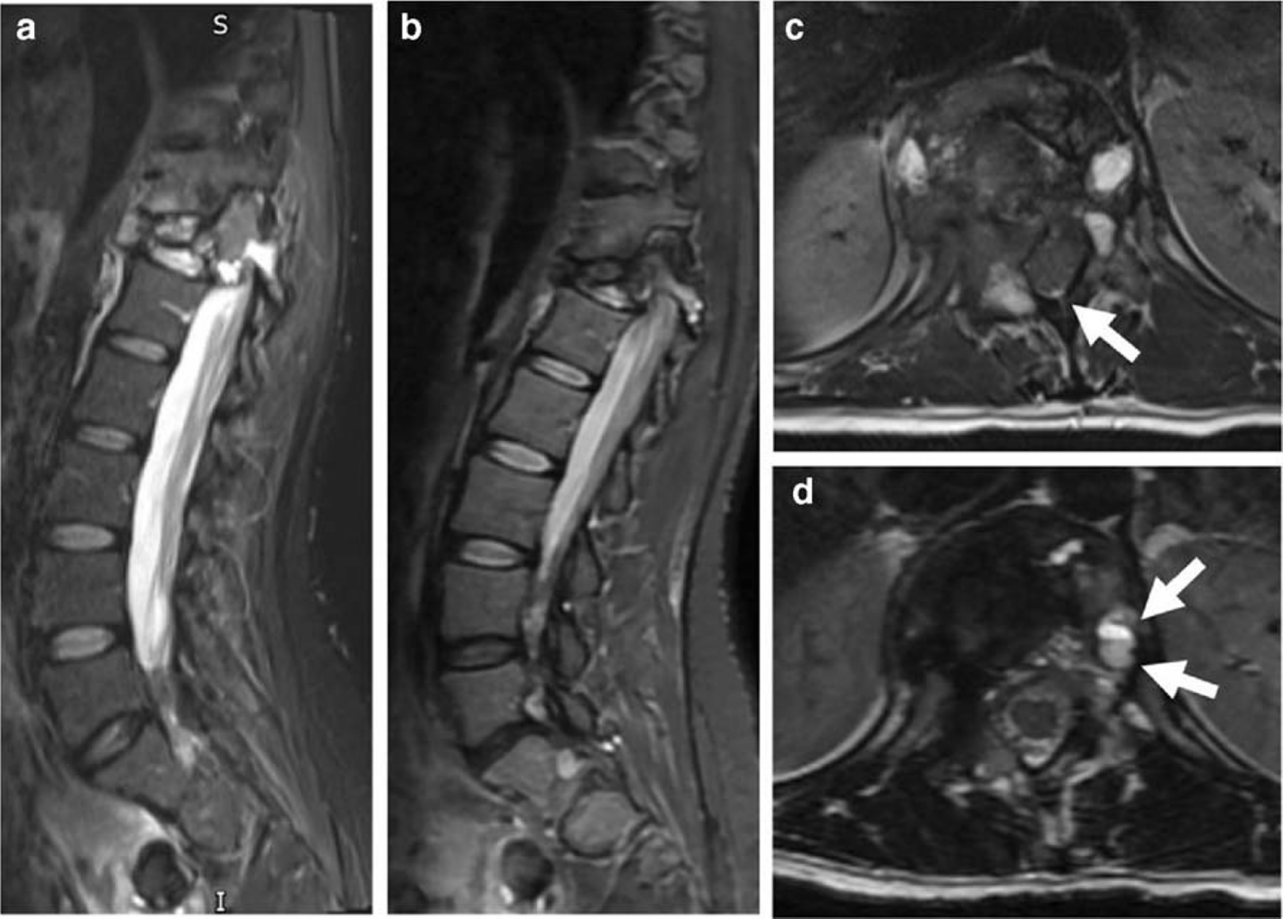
A 30-year-old female presented with back pain and a newly developed scoliosis. MRI at presentation (a, c) and after 8 weeks on denosumab (b, d). a Sagittal T2 TIRM MR image at baseline shows T12 vertebral collapse secondary to a GCTB with heterogeneous signal intensity and cystic components. b Sagittal T2 TIRM MR image after 8 weeks on denosumab demonstrates decreased size and signal intensity of the tumour, with further loss of height of the vertebral body. c Axial T2-weighted MR image at presentation shows tumour extension into the paravertebral soft tissues and epidural space. There is almost complete obliteration of the spinal canal with minimal remaining CSF posteriorly (white arrow). d Axial T2-weighted MR image after 8 weeks on denosumab shows decreased size and signal intensity of the tumour, with a more defined low signal margin and capacious spinal canal. One of the cystic components shows a fluid-fluid level, likely after haemorrhage (white arrows)
